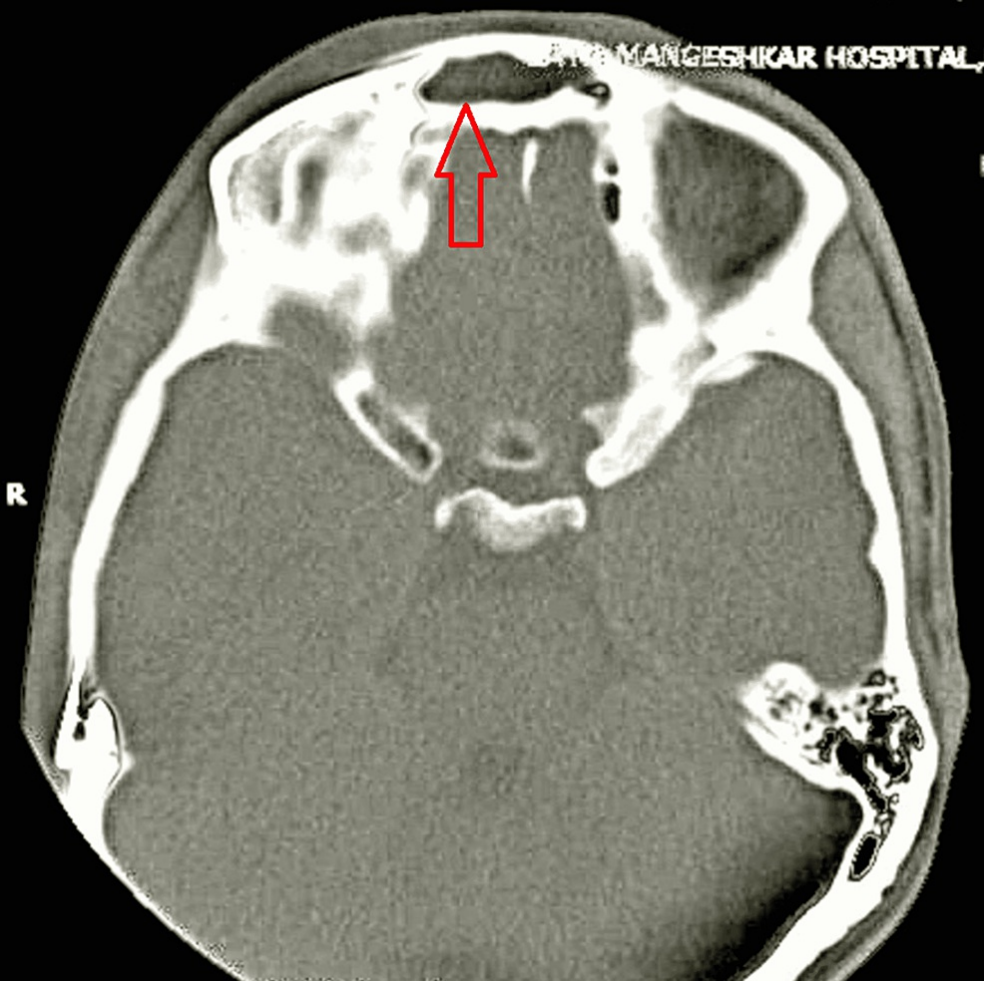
Axial plane CT-PNS image showing soft tissue density lesion completely occluding frontal sinus. PNS - paranasal sinuses
